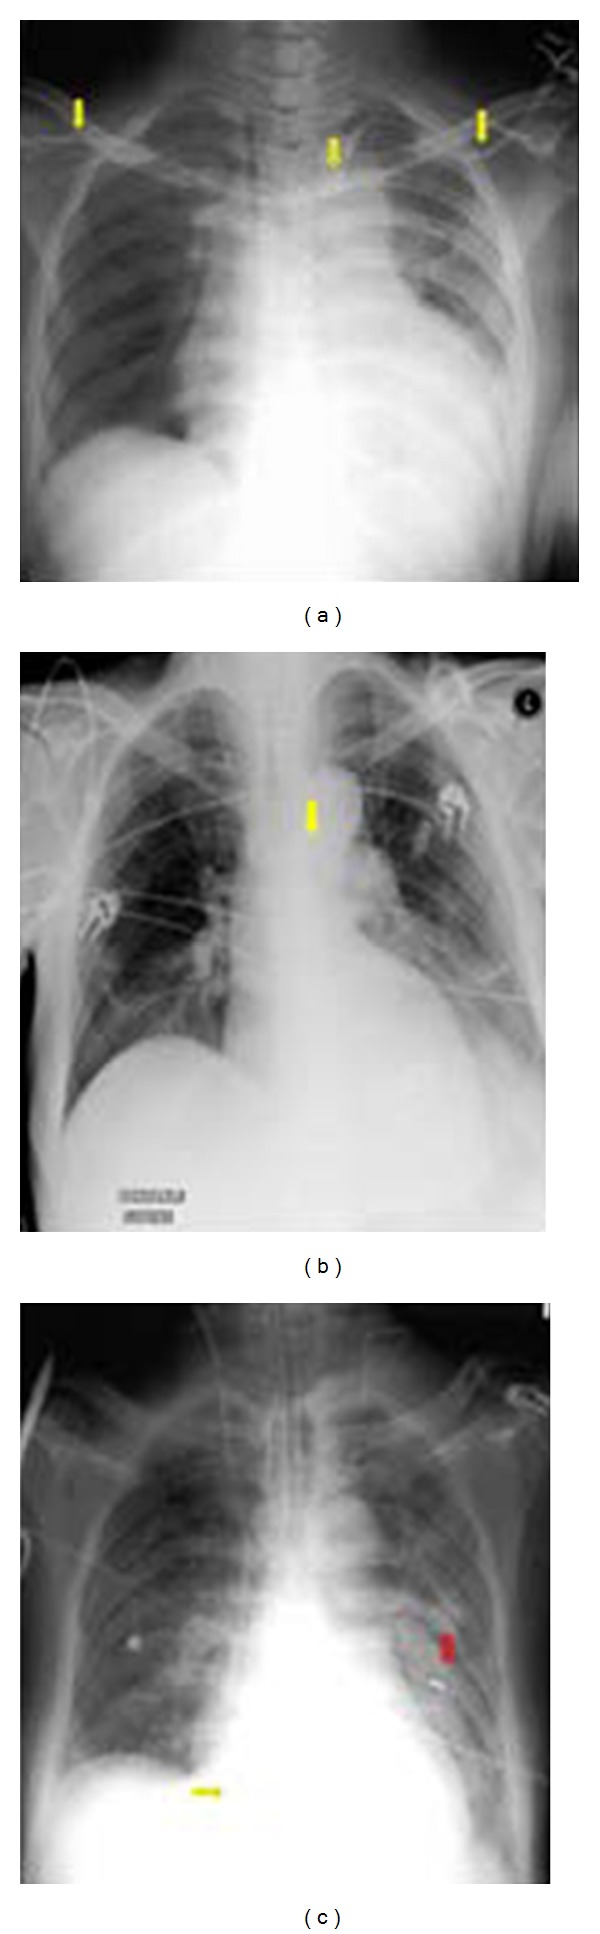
Portable AP radiographs on three different patients showing malpositioned central venous lines. (a) It shows that the IV line has crossed from the left axillary to the right axillary vein (arrows). (b) It shows the central line placed via the right jugular vein has entered the left brachiocephalic vein (arrow). (c) It shows that the tip of the central line has entered the inferior vena cava (yellow arrow), whilst the NGT has entered the left lower lobe bronchus associated with a left basal pneumonia.
